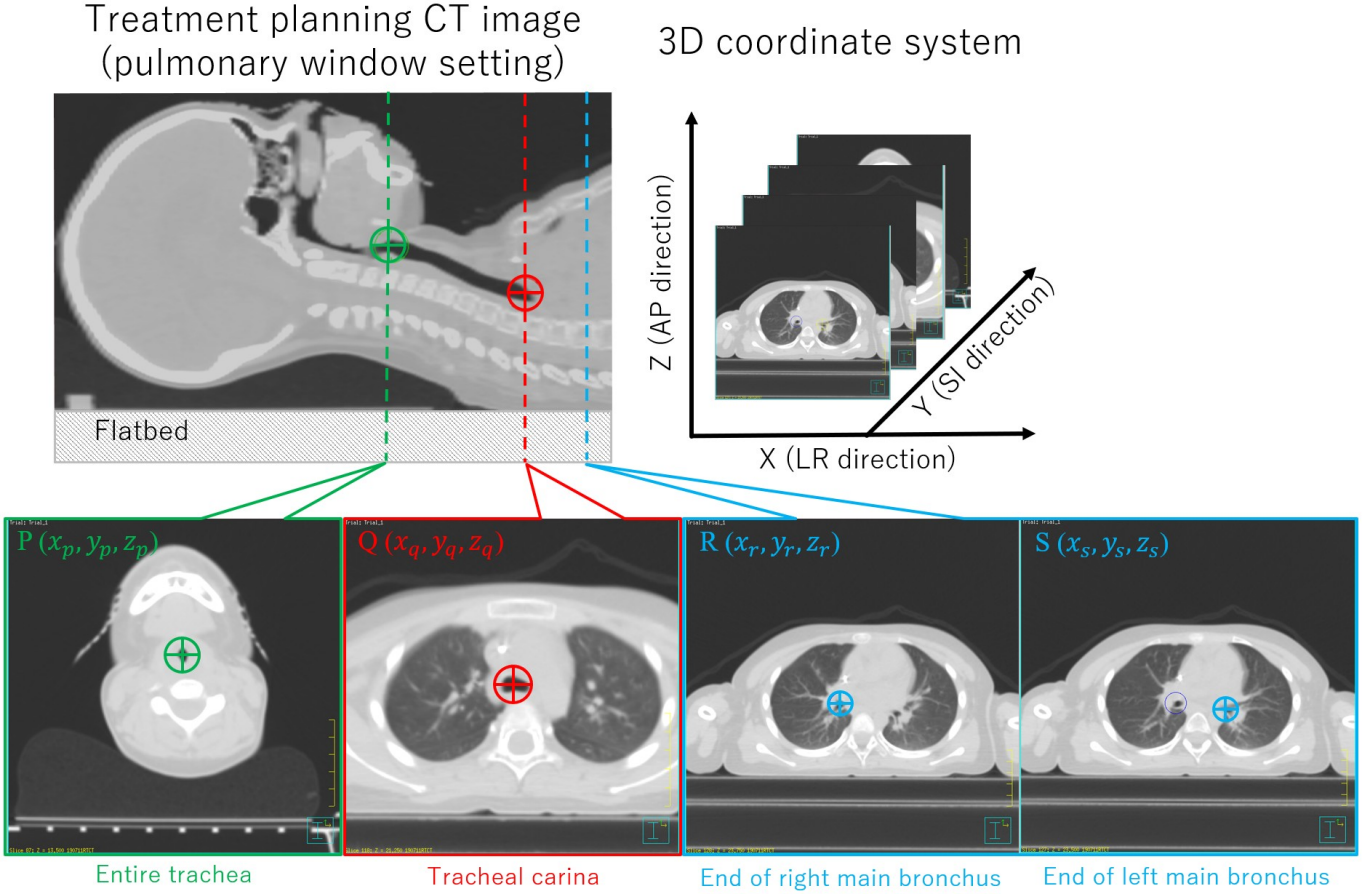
Measurement point in treatment planning CT images.
